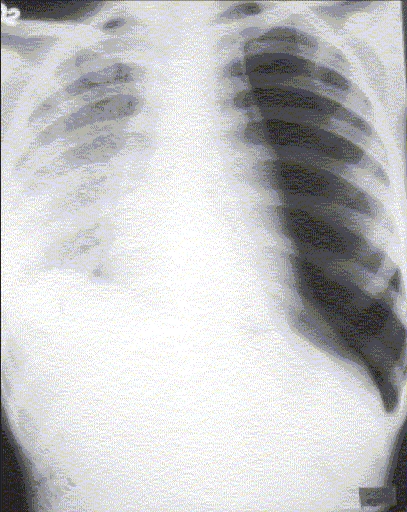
Chest radiograph frontal view showing left-sided pneumothorax.
